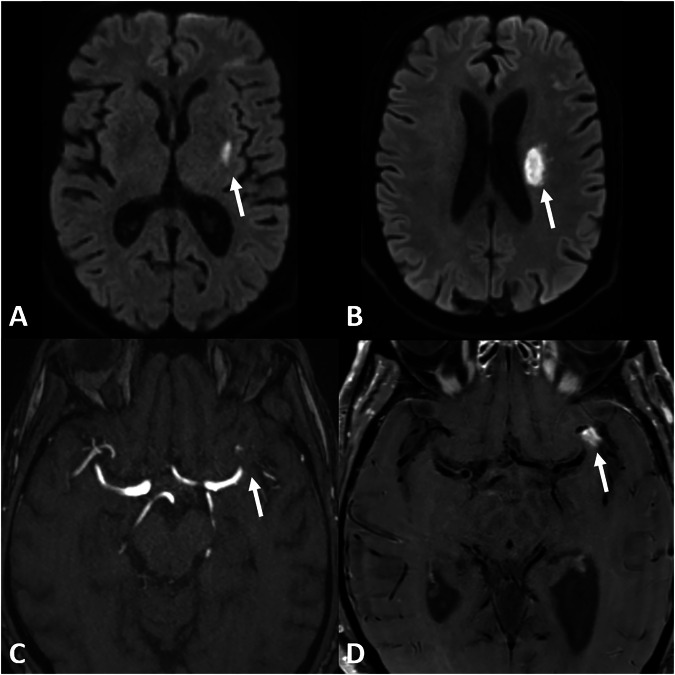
Initial imaging of a 74-year-old male patient who presented with right-sided hemiparesis and dysarthria. B1000 DWI (A, B) shows an infarction in the corona radiata left (arrow). There is a high-degree stenosis of the left MCA M1/M2 junctuion on TOF (arrow) (C) with focal vessel wall enhancement (arrow) (D) on T1 TSE dark blood. Therapy was initiated 1 day later with 1000 mg methylprednisolone for 3 days, followed by body weight-adapted prednisolone and cyclophosphamide
